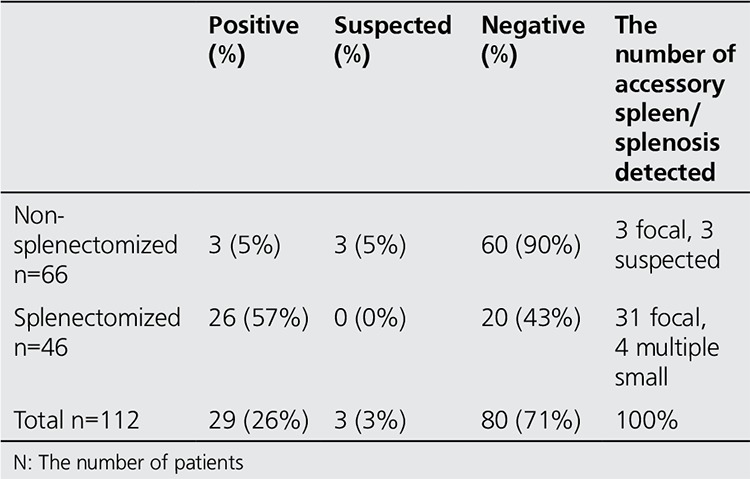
Distribution of the results of selective spleen scintigraphy in patients with and without splenectomy
